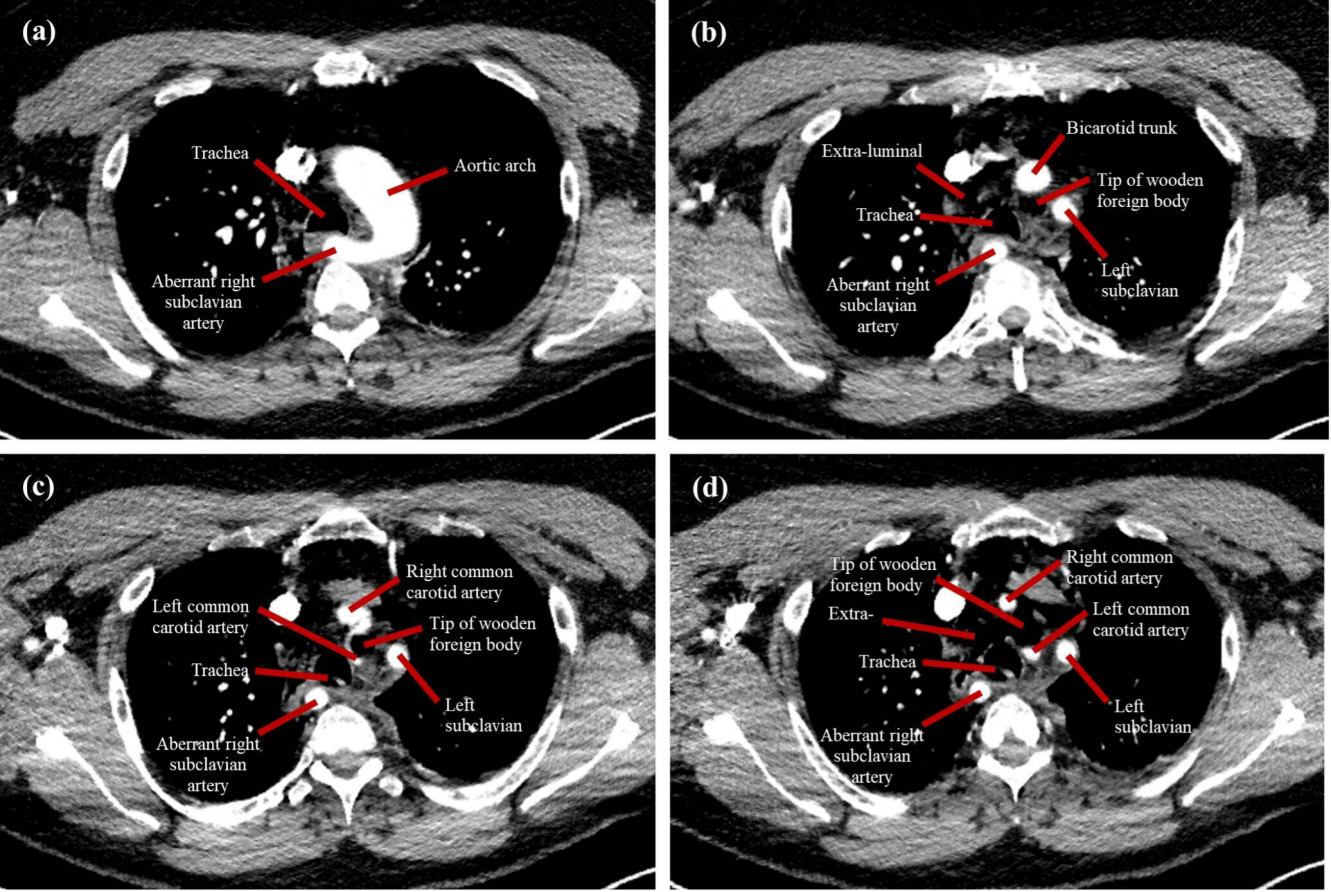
(a, b, c and d) Gallery of axial CTA images showing the pathway of the wooden foreign body through the major vessels of the aortic arch. Note the presence of the bicarotid trunk giving rise to the left and right common carotid arteries, as well as the retro-oesophageal course of the aberrant right subclavian artery. The left common carotid artery is visualised coursing around the medial aspect of the foreign body.
